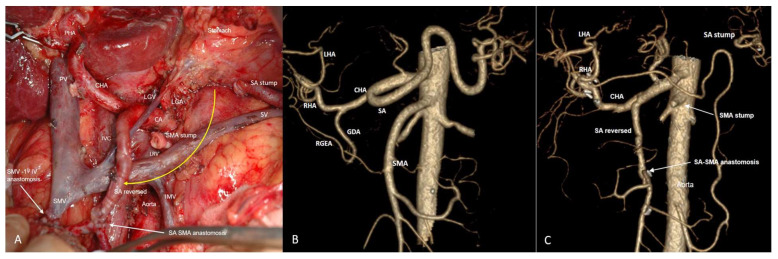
Total pylorus- and spleen-preserving pancreatectomy with SMA and SMV resection, resection and rotation of the splenic artery (SA) for the substitution of the SMA and SA-SMA anastomosis for LA PHDAC in a 73-year-old man. (A) An operating field after completion of the resection stage. Yellow arrow shows the clockwise rotation of the SA. (B,C) CTA before (B) and after (C) surgery, showing the postoperative sources of blood supply to the spleen from the branches of the LGA, the diameter of which increased by 1.6 times, increasing the VBF through the LGA by 7.4 times.
